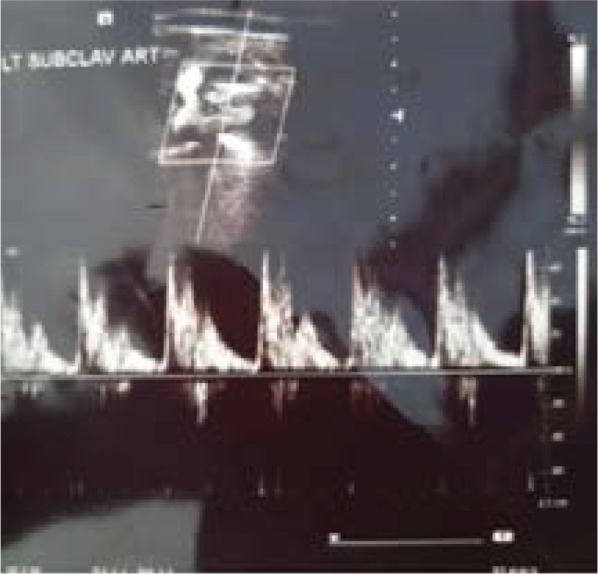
Left subclavian artery Doppler of the patient
